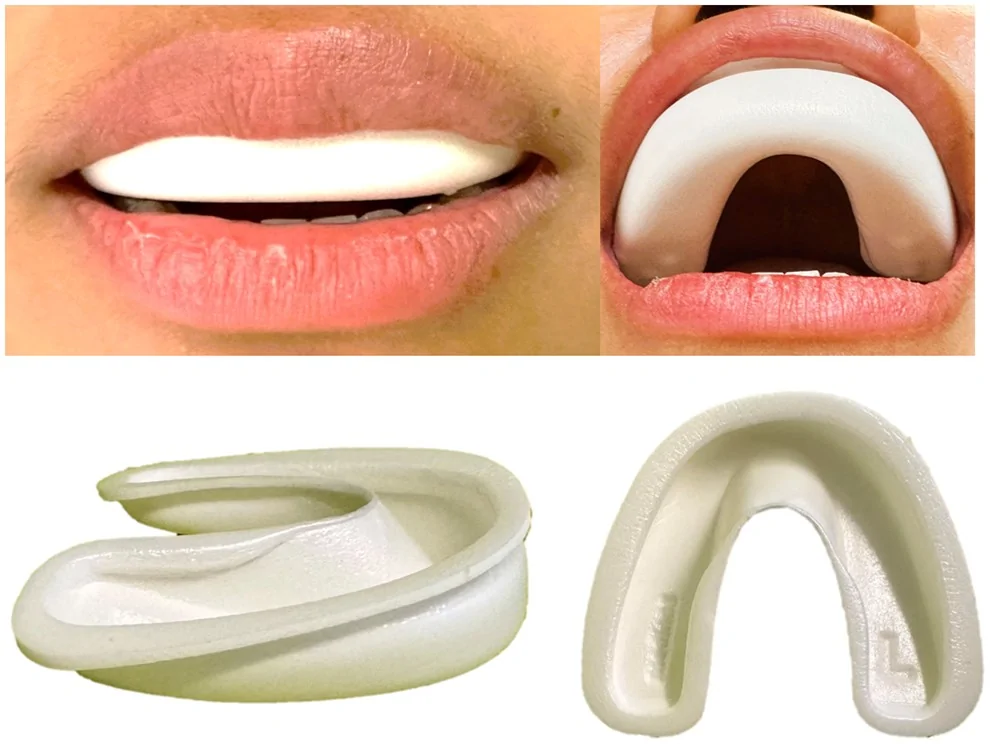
Fluoride tray with the intraoral view placed in the maxillary arch to aid soft tissue separation during CBCT scans.
